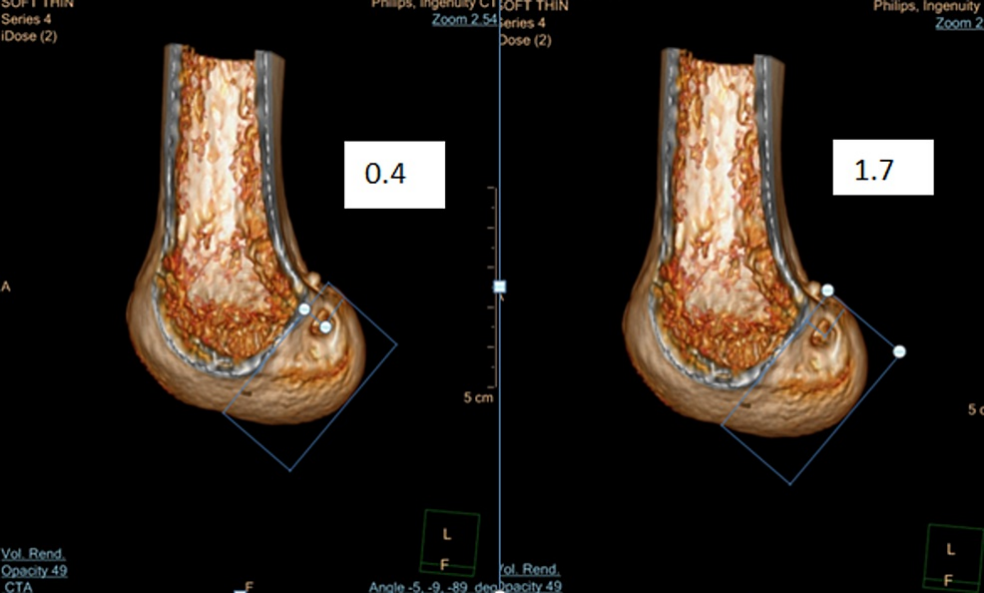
CT image of distal femur showing tunnel assessment in high-low position (left and right)
